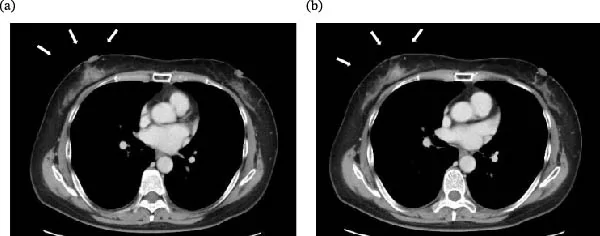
Computed tomography (a, b). Contrast‐enhanced computed tomography (CT) showed an area of ill‐defined borders and segmental faint enhancement immediately in the upper‐outer and upper‐inner quadrants of the right breast, corresponding to breast cancer. No lymph node or distant metastasis was observed.
